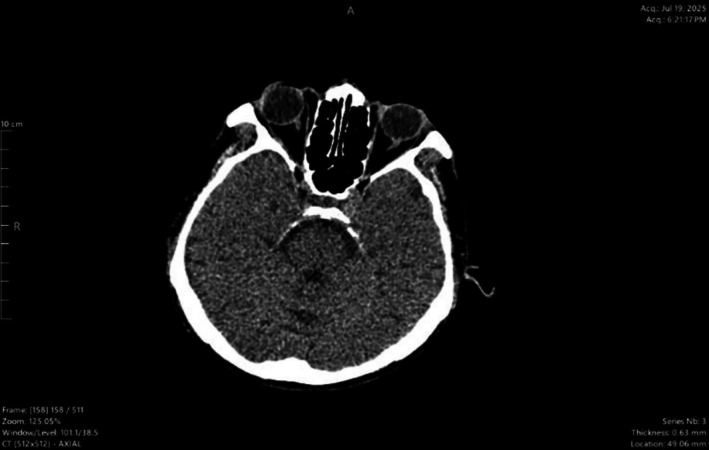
Urgent CT brain done at Day 9 when suspecting in—hospital stroke—showing no infraction or ICH (intracranial hemorrhage).
